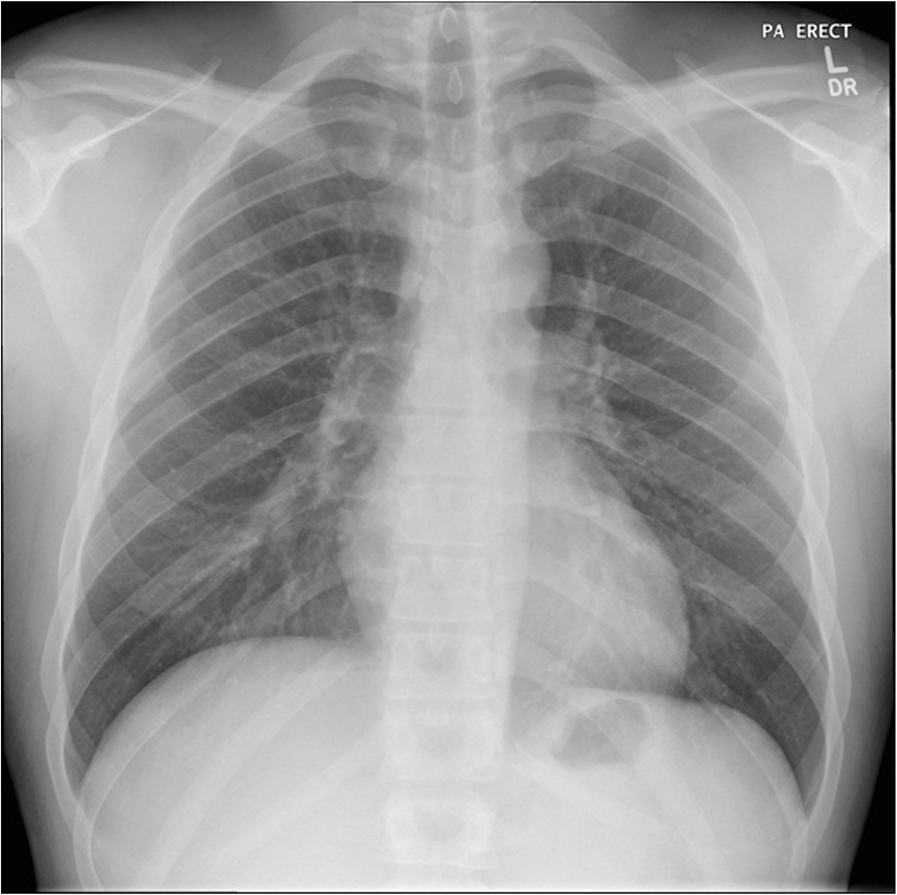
Follow up chest x-ray shows considerable reduction in the soft tissue previously noted in relation to the right paratracheal stripe
